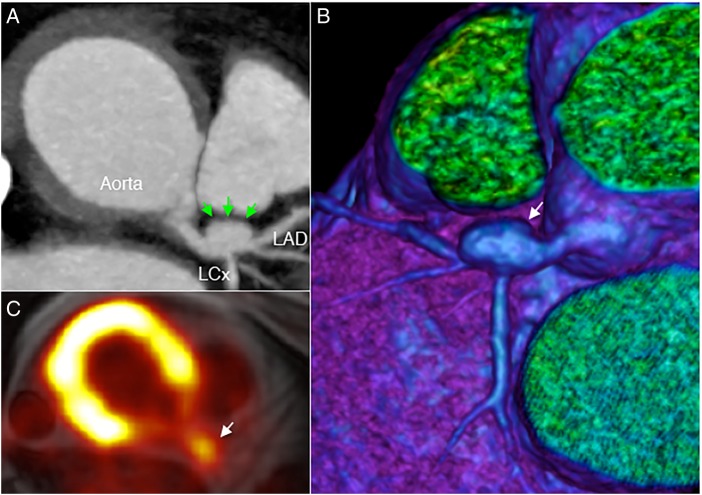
Coronary artery aneurysm (arrows) shown using CT angiography (A), three-dimensional CT reconstruction (B) and ^18^F-fluorodeoxyglucose positron emission tomography-MRI (C). LAD, left anterior descending artery; LCx, left circumflex artery.
